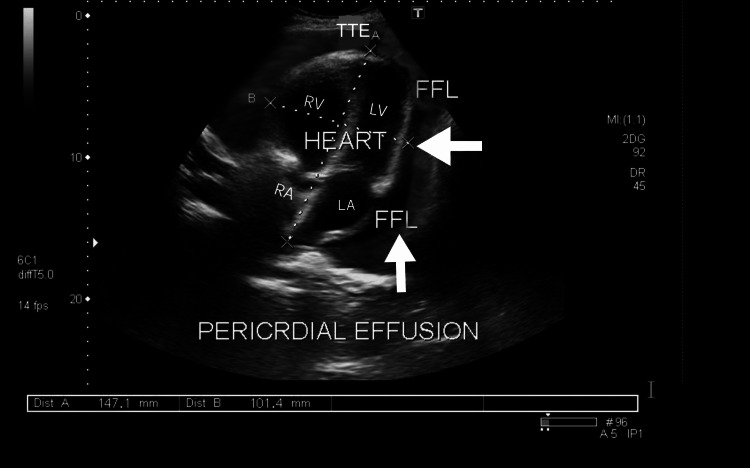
Transthoracic echocardiography (TEE) Subcostal View Showing Pericardial Effusion.
